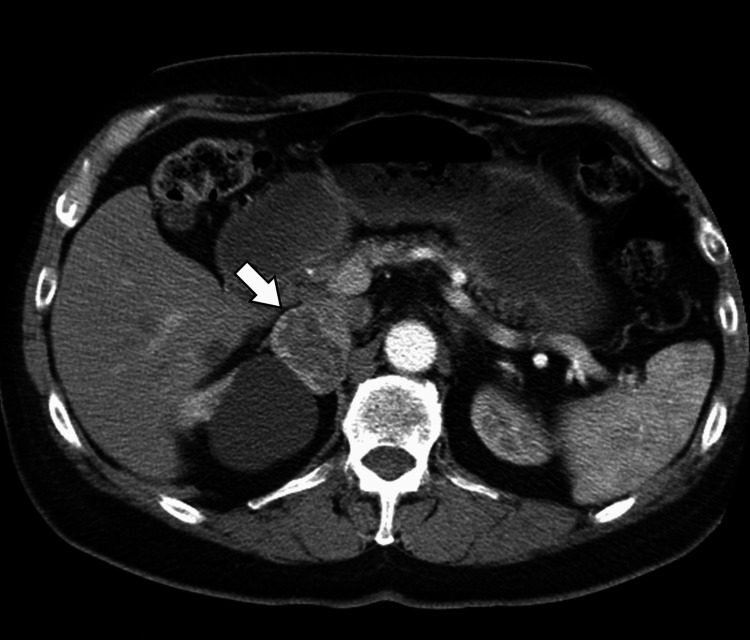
Contrast-enhanced axial CT of the abdomen Axial contrast-enhanced computed tomography (CT) image shows a large, heterogeneous soft tissue lesion in the right adrenal gland (arrow) with areas of low attenuation and mild peripheral enhancement.
